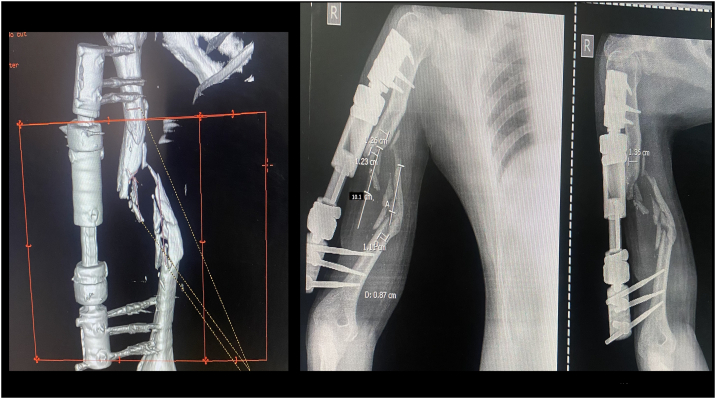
Patient presented to us three months post initial procedure, CT scan and X-rays of right arm depicted a 10 cm segmental defect of the humeral diaphysis with an external fixator in-situ.
